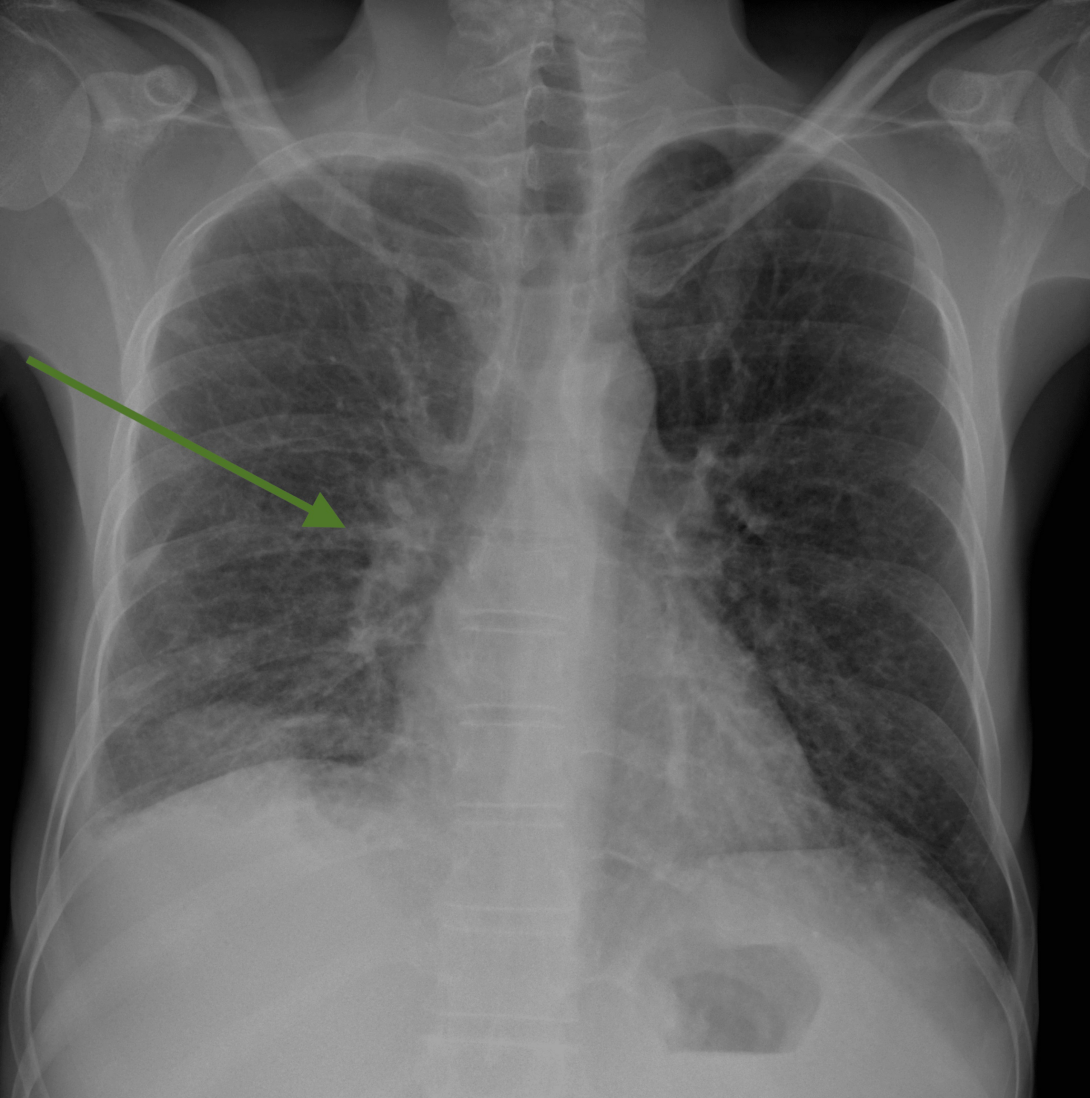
Chest X-ray showing mediastinal lymphadenopathy (green arrow), with pulmonary nodules and right-sided pleural effusion
